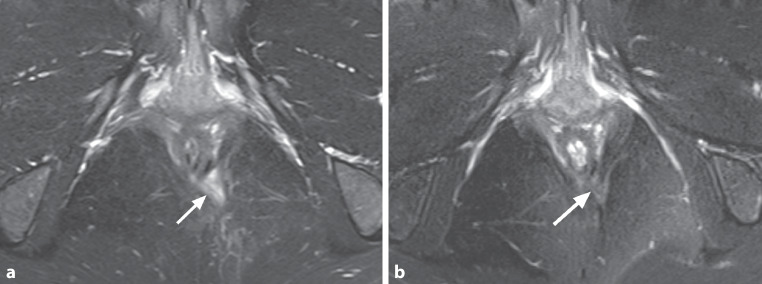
MRI of a 32-year-old female patient with perianal fistula. a Axial T2-weighted fat-saturated image before surgery shows a left-sided transsphincteric fistula with high signal (*arrow*), proving the fluid component within the fistula. b The fistula tract 10 months after surgery shows a dark signal on T2-weighted fat-saturated axial image (*arrow*), indicating fibrosis and response to treatment
